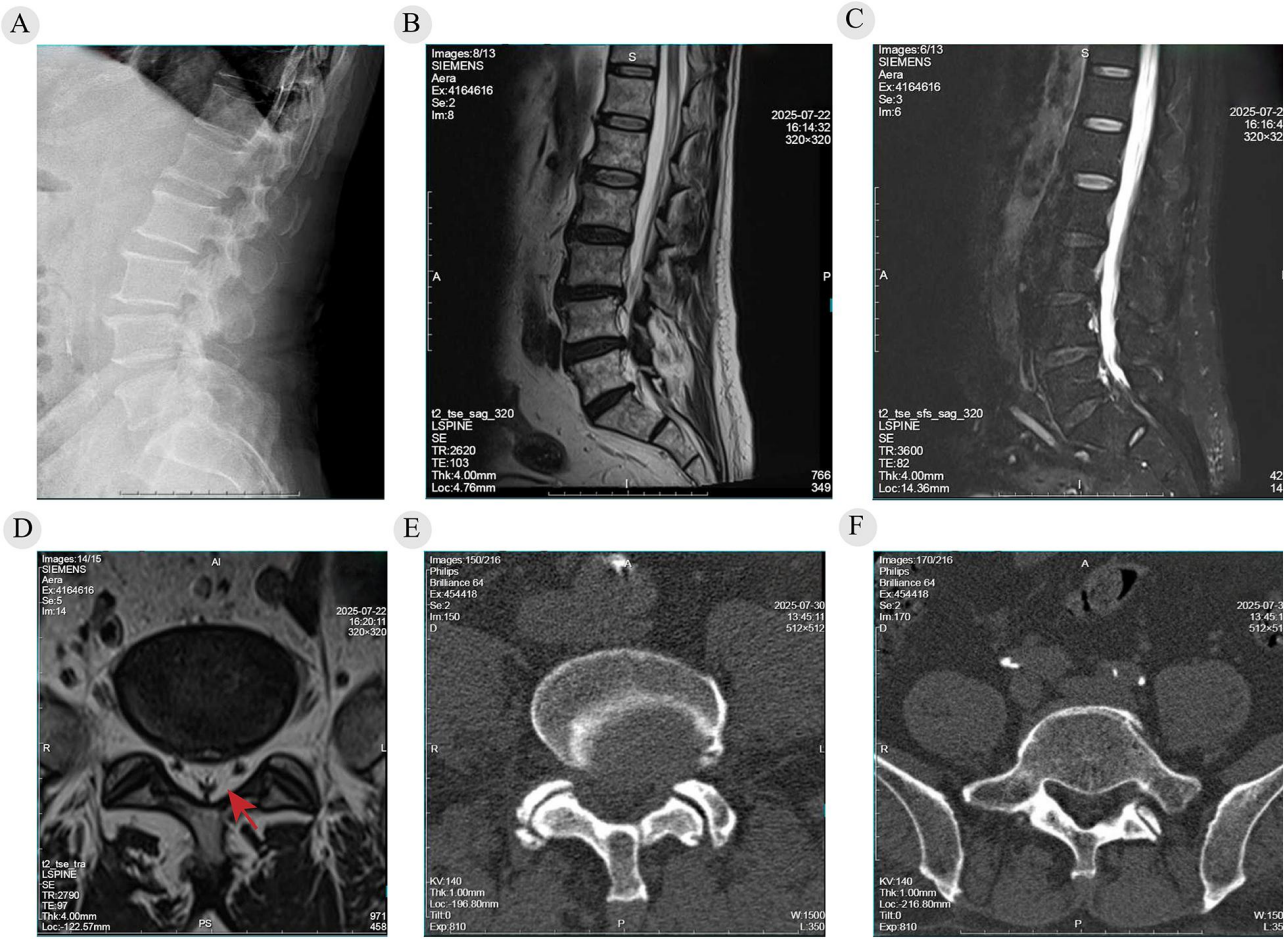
Preoperative imaging findings and key features of LSS complicated by SEL. (A) Lateral lumbar radiograph showing degenerative disc disease with reduced intervertebral disc height. (B) Sagittal T1-weighted MRI revealing central canal stenosis at L4–L5 and mild disc protrusion at L3/4 and L4/5. (C) Sagittal T2-weighted fat-suppressed MRI demonstrating marked posterior epidural lipomatosis from L4 to S1 and ligamentum flavum hypertrophy at L4/5. (D) Axial T2-weighted MRI at L5/S1 showing severe circumferential compression of the thecal sac by excessive epidural fat, forming the typical “Y-sign” (red arrow). (E) Axial CT at L4/L5 showing degenerative osseous changes without calcification. (F) Axial CT at L5/S1 confirming no calcification and outlining the lumbosacral osseous canal morphology. Written consent for publication was obtained.
